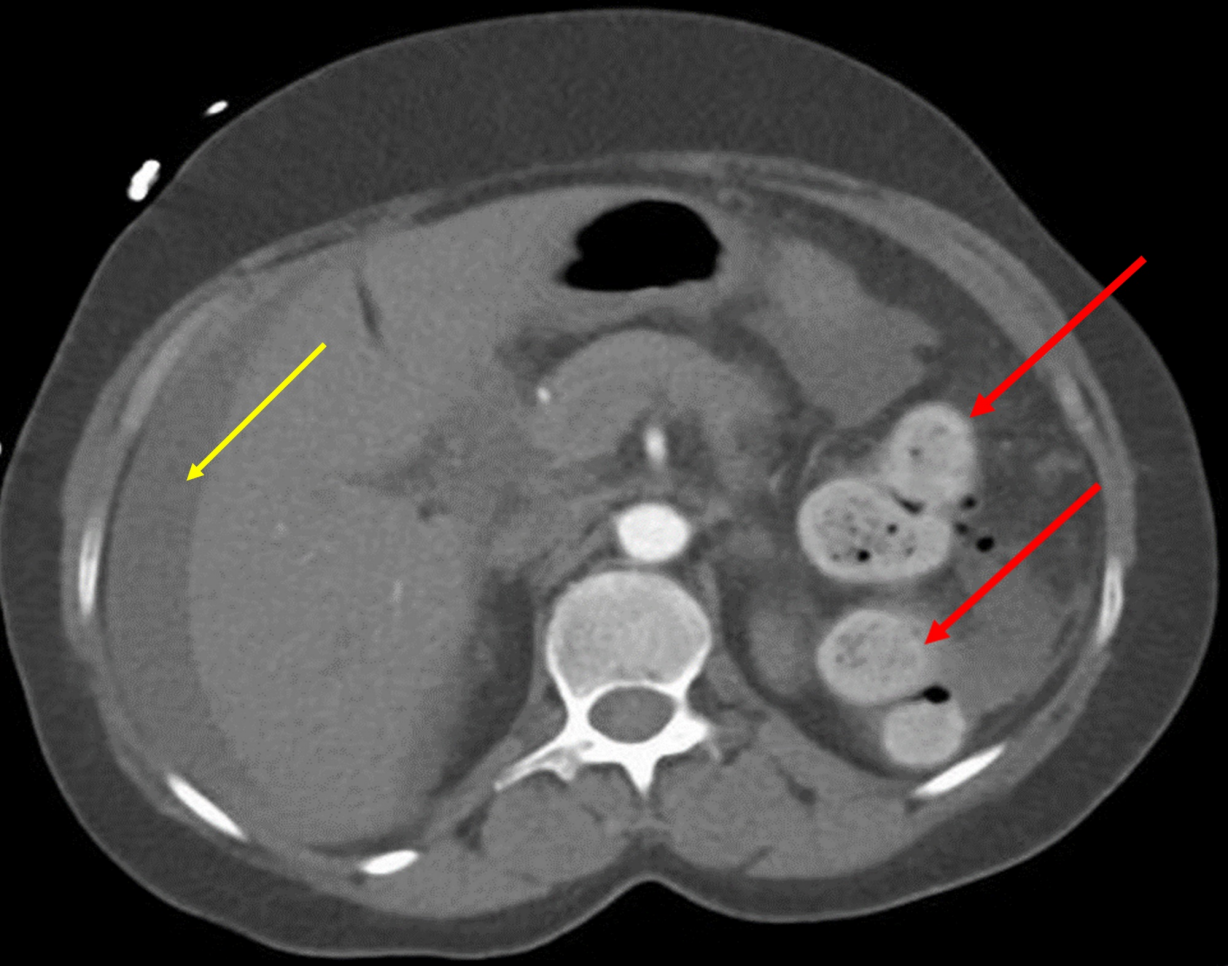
Non-enhanced CT scan of the abdomen, axial view Left colon loaded with fecal matter and indicated by red arrows. Free fluid in the peritoneal cavity is shown with yellow arrow
